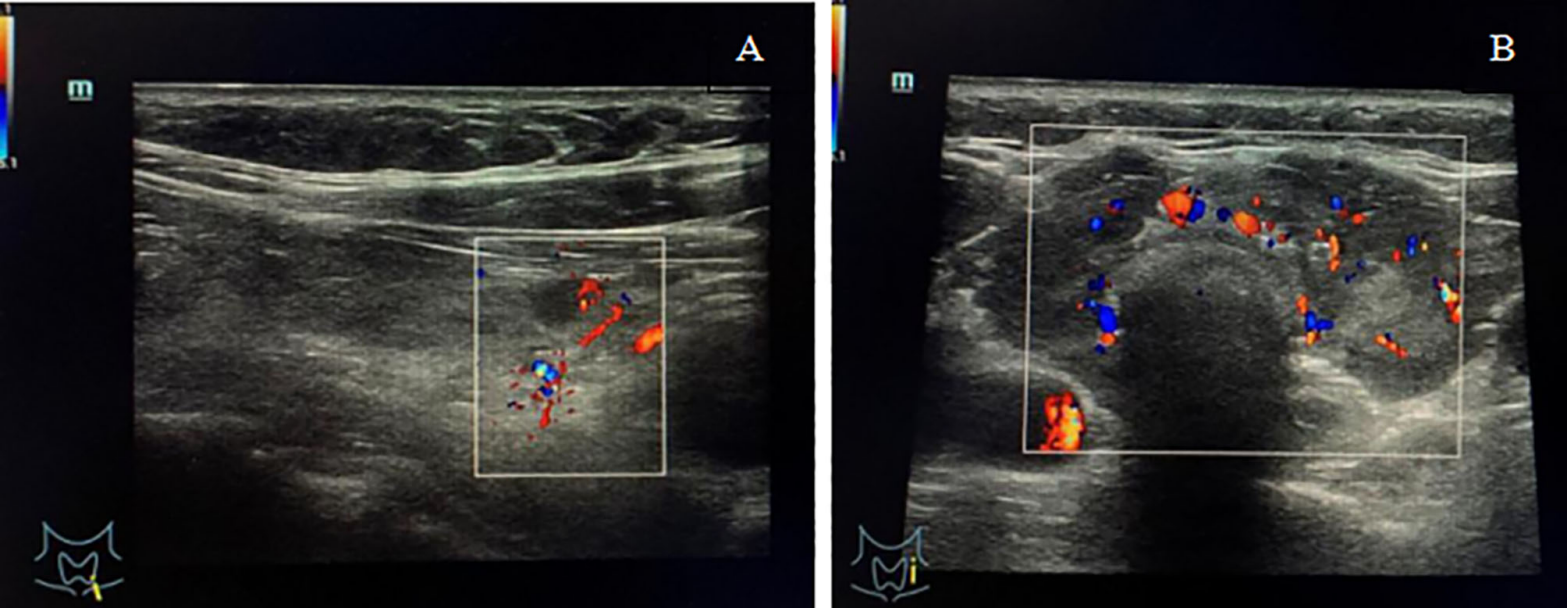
(A) Ultrasonography showed bilateral thyroid reduction and multiple nodules (<0.5 cm in diameter) in the left lobe with normal blood flow signals. (B) Ultrasonography showed thyroid enlargement with diffuse reduction in gland echo and abundant blood flow signals.
